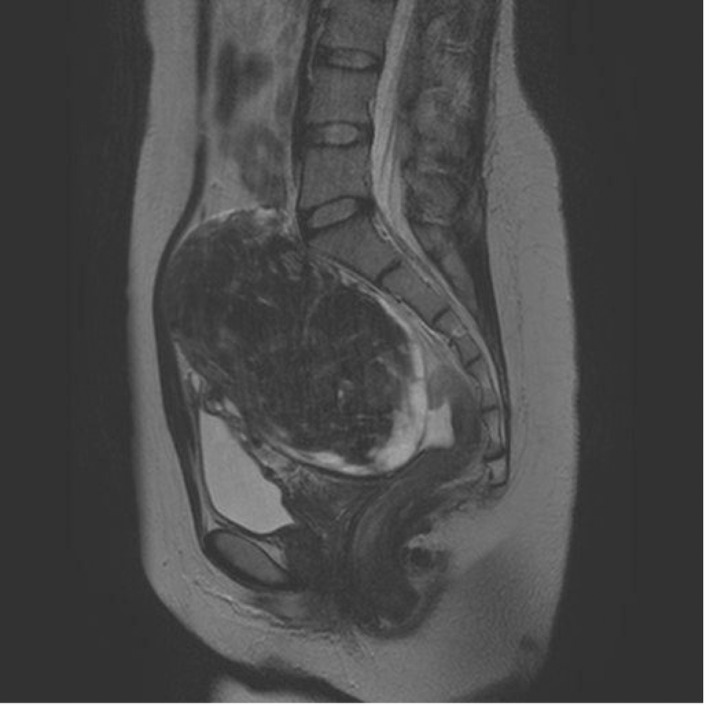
Abdominal MRI of case 1. An MRI scan revealed a UL 13 cm in diameter. Although the pelvic plexus itself could not be visualized, the size and the posterior‐base location of the tumor was thought to compress the pelvic plexus in this patient.
